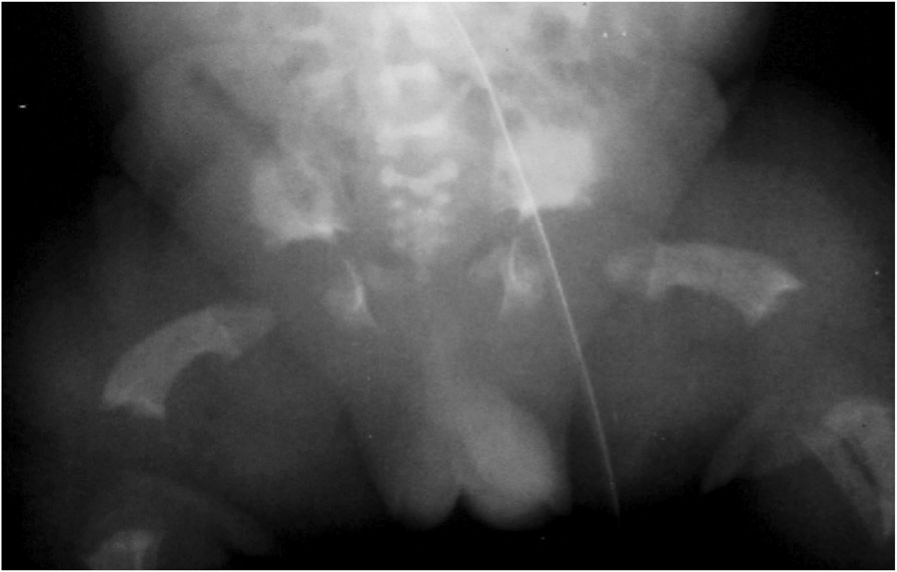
Anteroposterior radiograph of the pelvis and femora in thanatophoric dysplasia, type I. Here, too, qualitatively most of the abnormal characteristics are similar to those seen in achondroplasia, but quantitatively all of them are much more severe. Note the so-called telephone receiver femora
